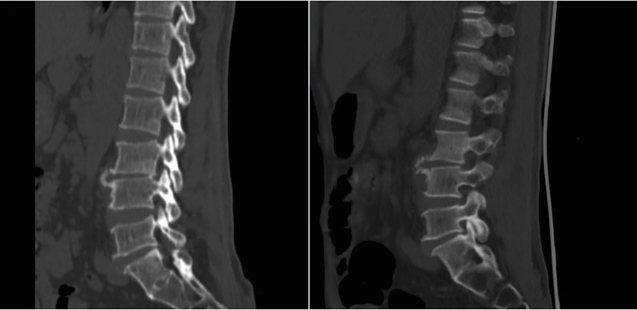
Computed tomography scan showing apparent destruction at the anterior superior corner of the L4 vertebra (Pedro Pons’ sign) accompanied by prominent osteosclerosis and osteophytes resembling a parrot’s beak.
